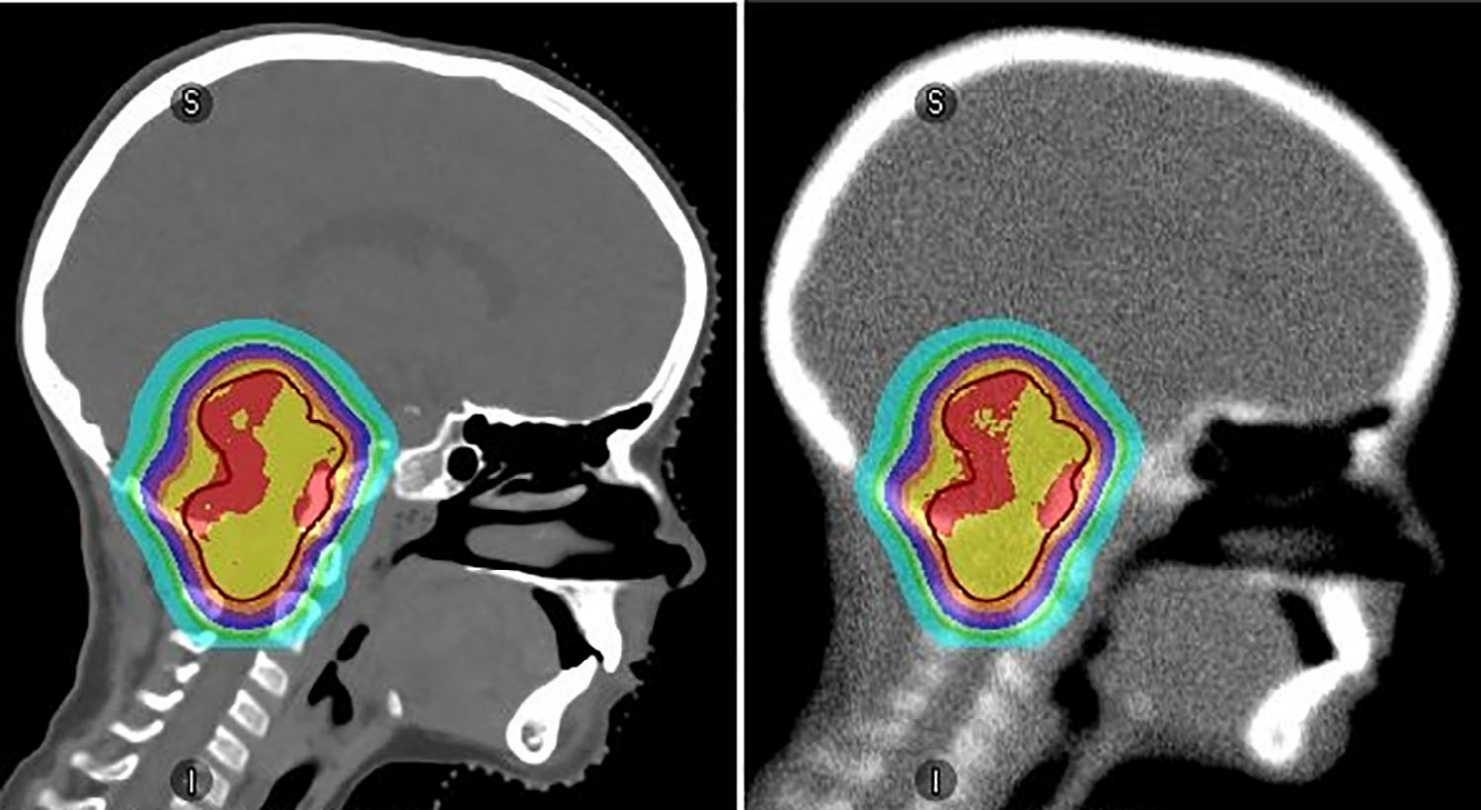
Exemplary sagittal dose distributions (colorwash overlay with the same color code as Figure 2) of patient ID 2. The red contour indicates the PTV. Left: planning CT overlaid with clinical dose distribution. Right: Modified CT (folded with Gaussian of width = 4.7 mm [FWHM] and applied noise of σ = 48 HU). The dose distribution computed on this CT is equivalent to the left one with a gamma pass rate of 98.9% (1 mm/1%). CT, computed tomography; FWHM, full‐width‐at‐half‐maximum; HU, Hounsfield unit; PTV, planning target volume.
